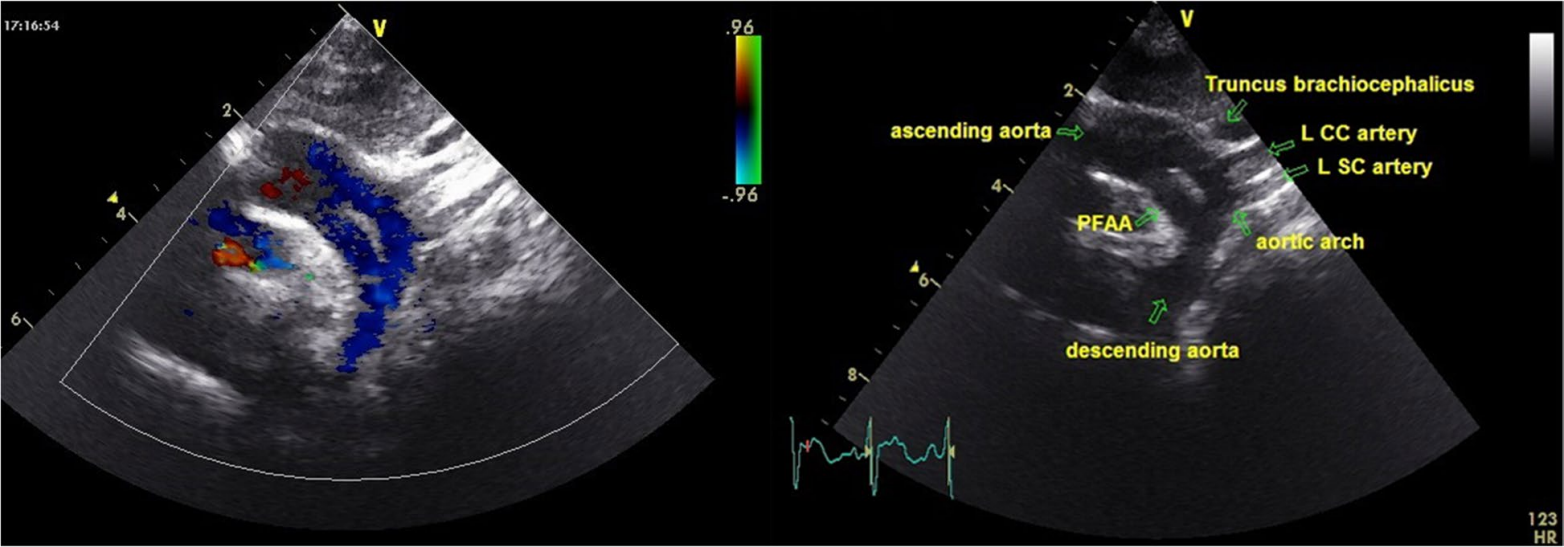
Persistent fifth aortic arch; echocardiographic 2D and color doppler view
